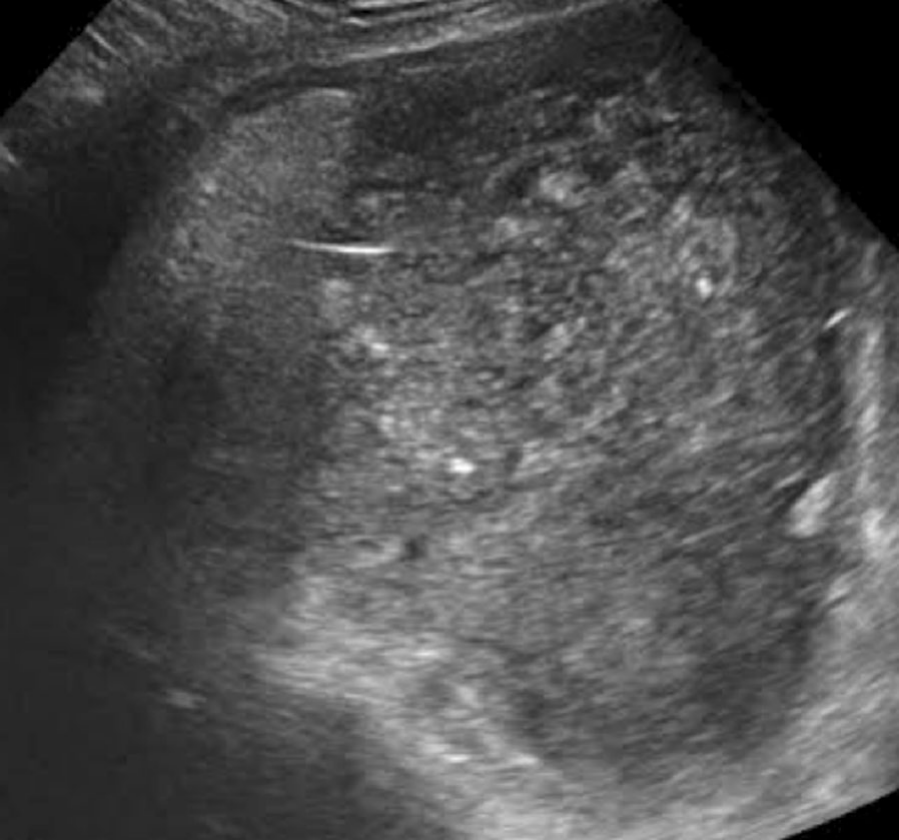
Transvaginal ultrasound image. A pelvic mass is seen of approximately 11 cm in diameter and is suspected to contain hair because of the presence of hyperechoic lines
